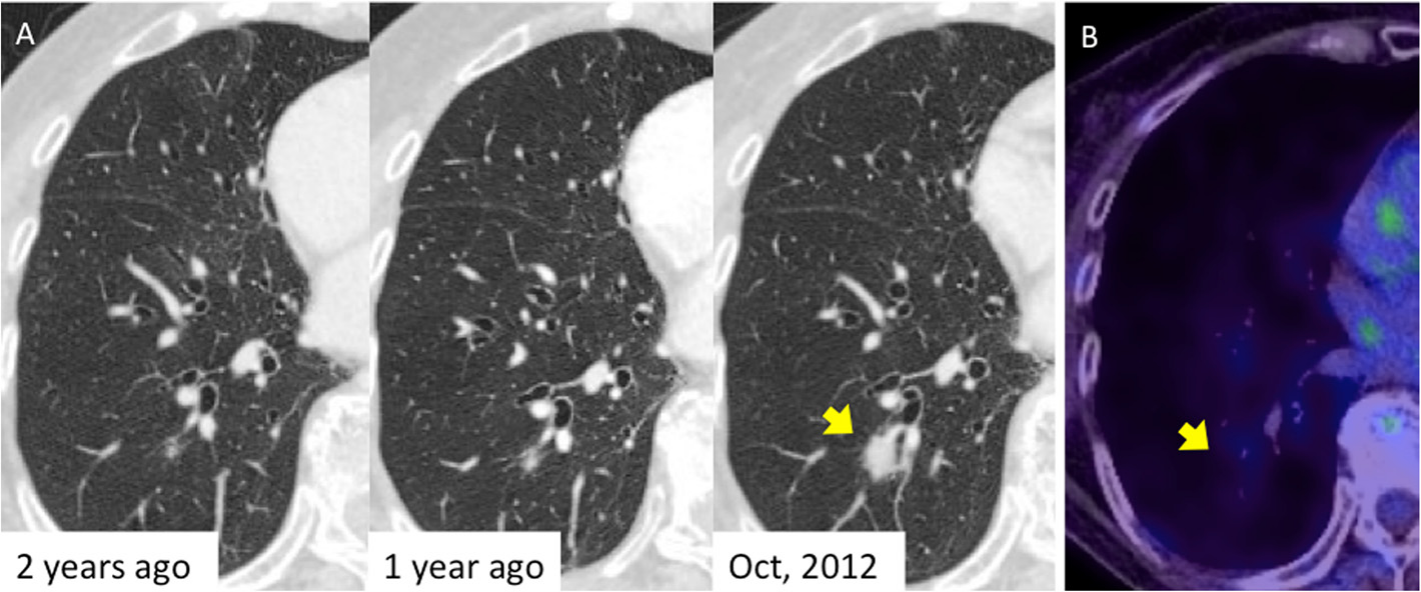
Radiological findings of the nodule. (A) Chest computed tomography (CT) shows an irregularly shaped nodule in the right lower lobe. A tiny nodule at the same site had been found two years earlier. The nodule had since shown a clear tendency to increase in size. The arrow points to the lesion. (B) Positron emission tomography (PET)-CT shows slight accumulation of fluorodeoxyglucose in the nodule (SUVmax = 1.5). The lesion is indicated by the arrow.
